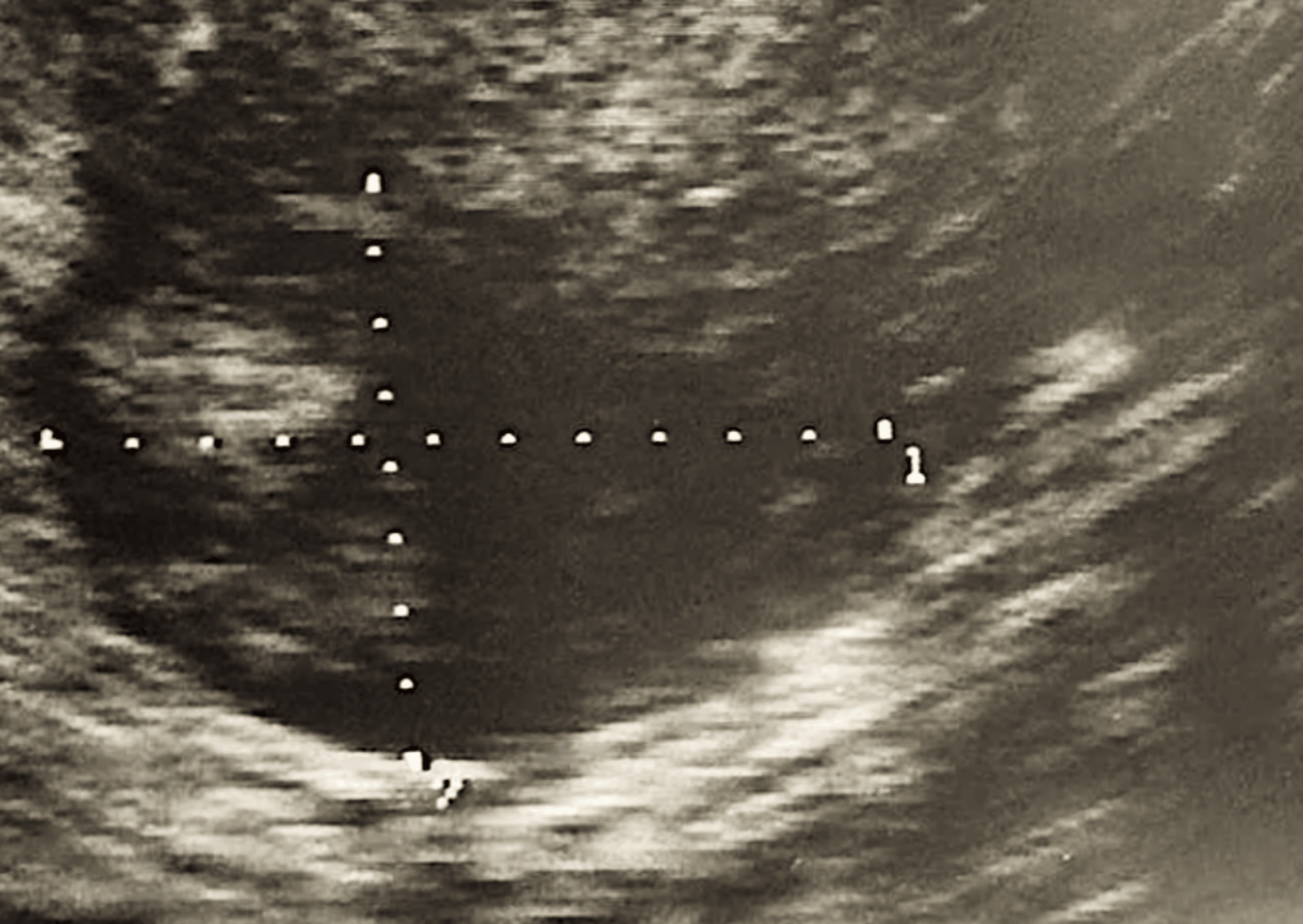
Ultrasound view of the left adnexal area based on the suspicion raised during the first surgery
